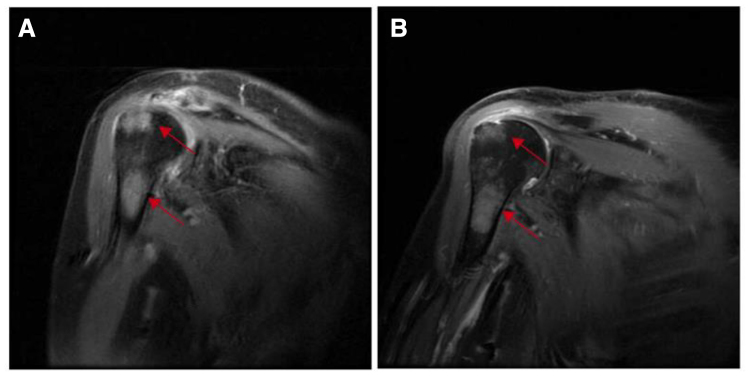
MRI of the right humeral head and neck at 4 months (A) and 6 months (B) after the initiation of antituberculosis therapy. The images show progressive attenuation of the abnormal signal and obvious regression of the lesion over time.
